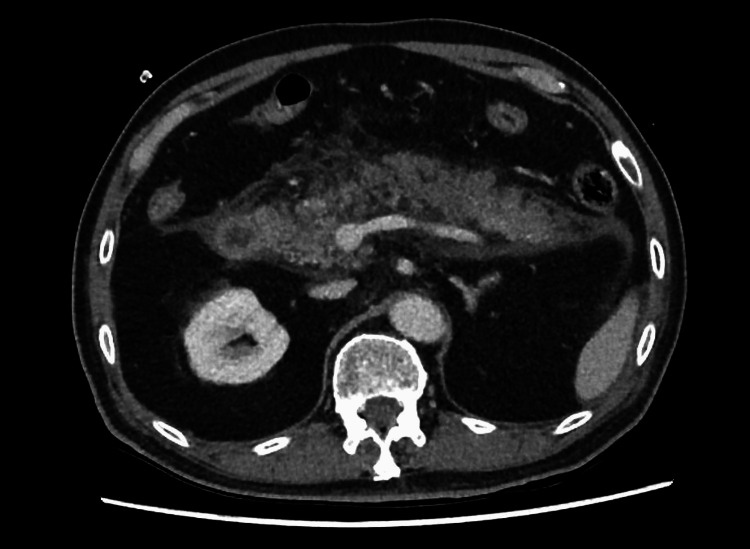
Contrast-enhanced CT in the portal venous phase, done on admission, showing hypoenhancing regions in the pancreatic neck and body
